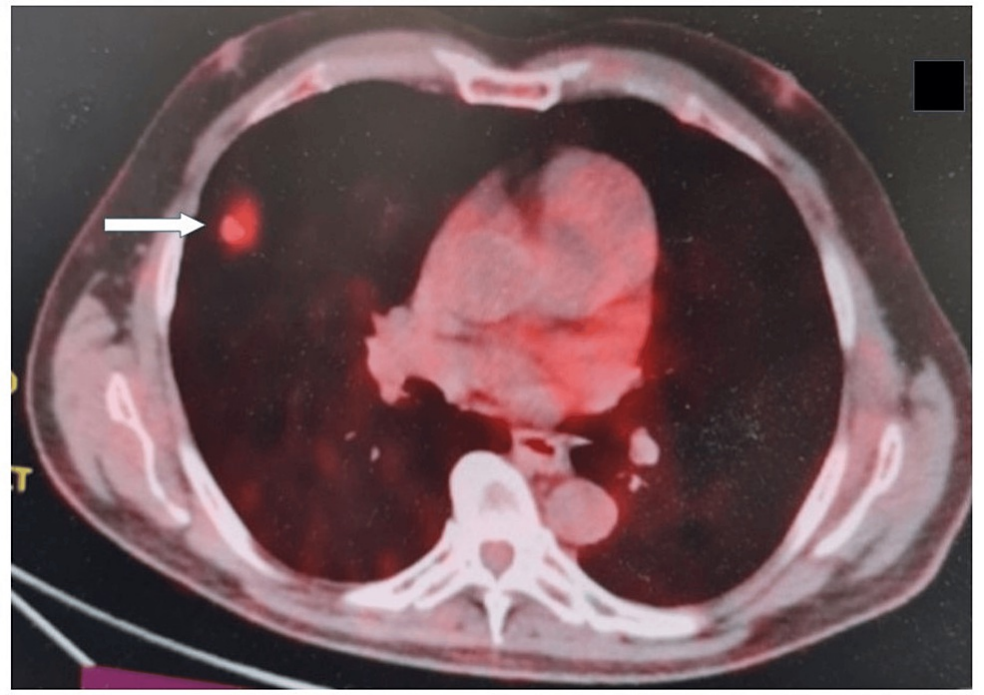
Preoperative PET/CT scan. The standardized uptake value of the atypical carcinoid (arrow) was 4.9.
